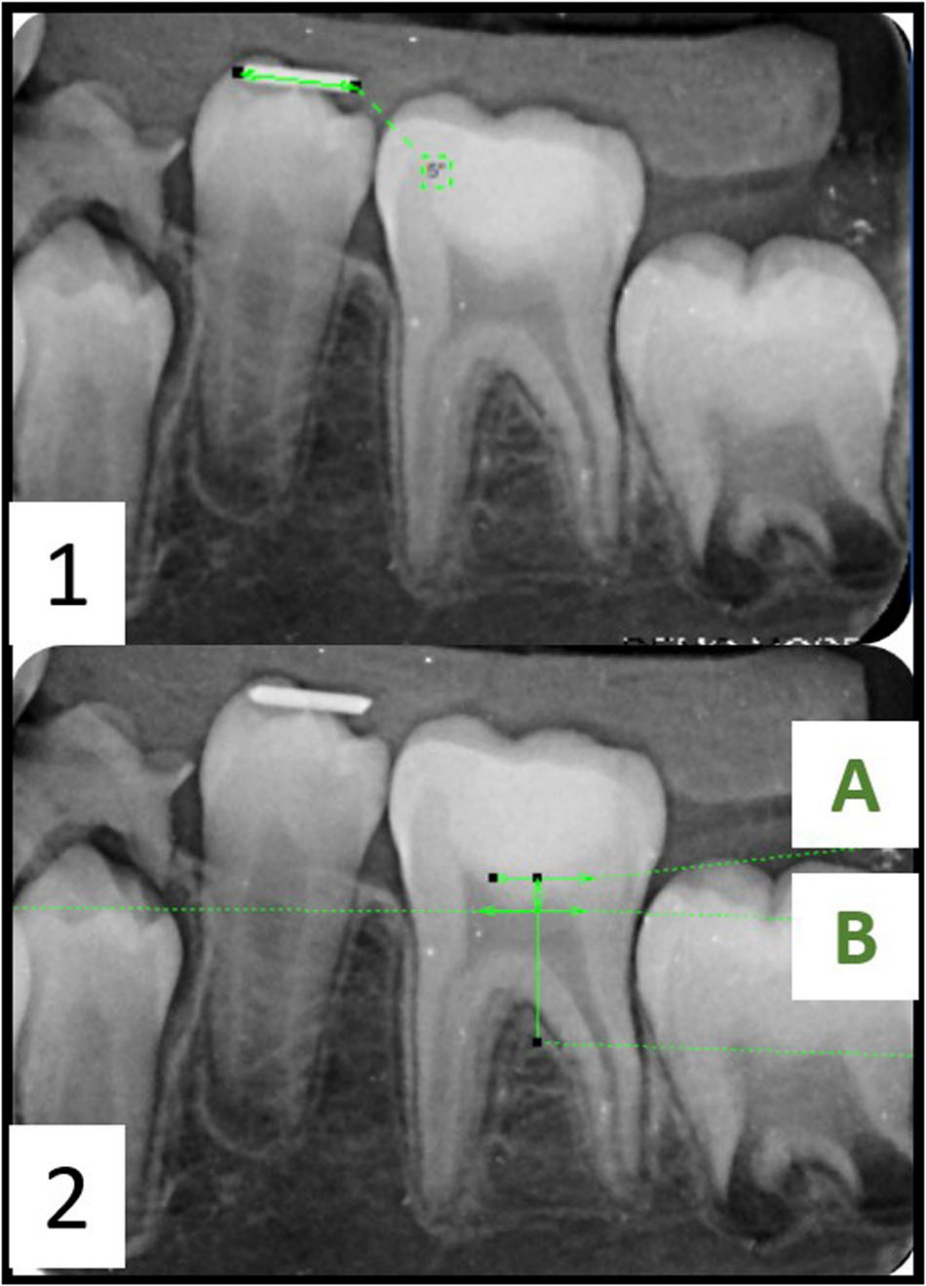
Dentin bridge measurement; (1) Postoperative radiograph showing the wire used in the calibration step, (2) reference lines used in measurement; (A) Reference point representing the most apical point of the restoration, (B) Reference point representing the most apical point in the dentin beneath the restoration corresponding to point A, (A, B) The distance between point A and point B is the line representing the remaining dentin thickness.
